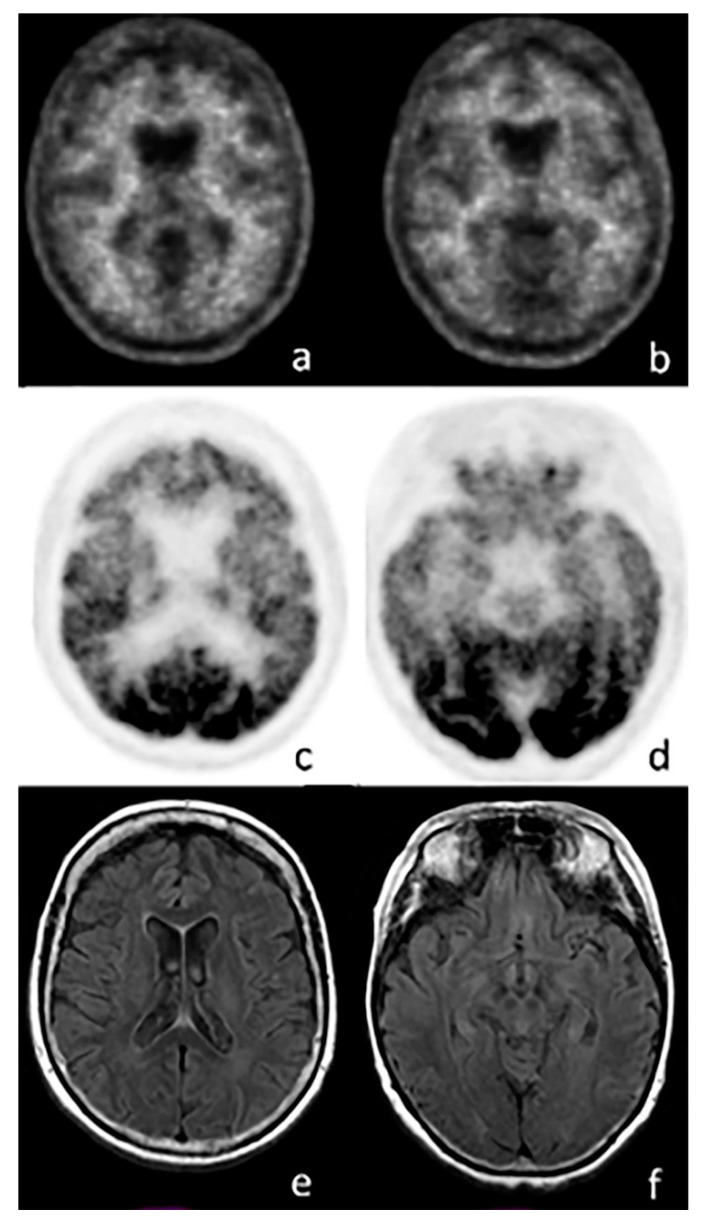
Axial images of a PET scan performed with ^18^F-FBB (a,b), ^18^F-FDG (c,d), and MRI (e,f) in a patient with suspected frontotemporal dementia (FTD). The images show no amyloid burden in the cortex, while a significant reduction in cortical glucose consumption is visible in the frontal (c) and temporal lobes (d). MRI does not show any abnormal findings.
